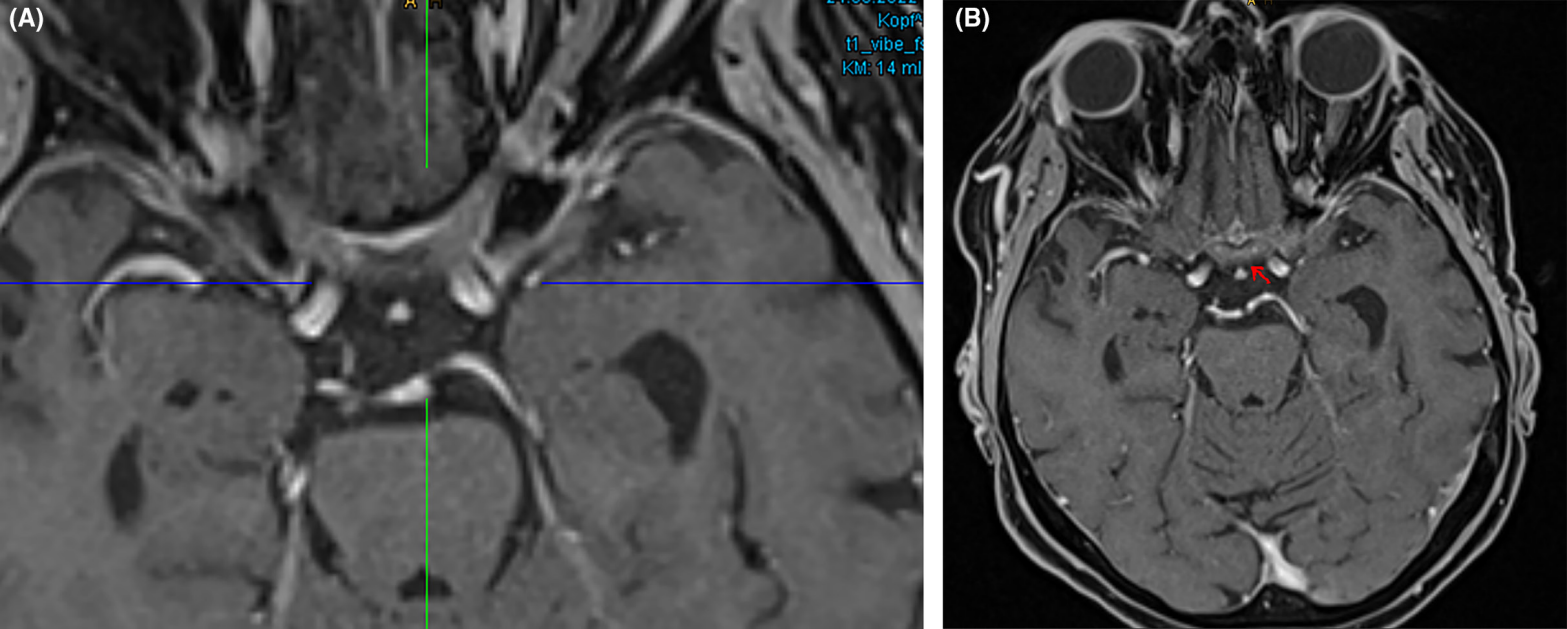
(A) Follow‐up MRI, axial T1 with contrast, showing no progress after tumor removal in the chiasma region. (B) Follow‐up MRI, axial T1 with contrast, showing no progress after tumor removal intracranially.
